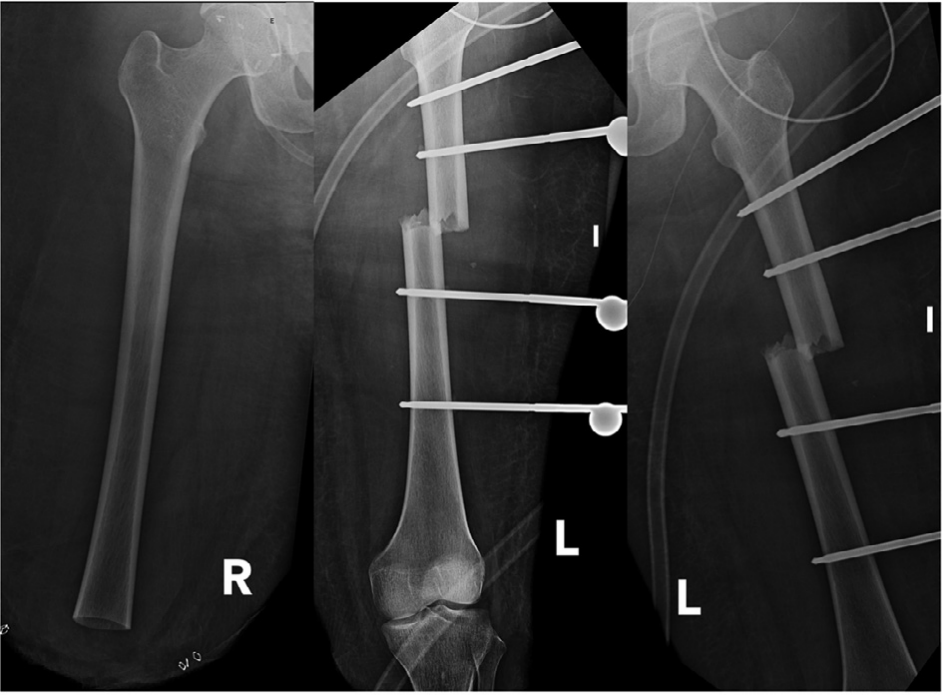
Femoral radiographs after damage control surgery. Right lower limb underwent a supracondylar amputation (left image). Left lower limb underwent a transtibial amputation and a femoral external fixation (middle and right images).
